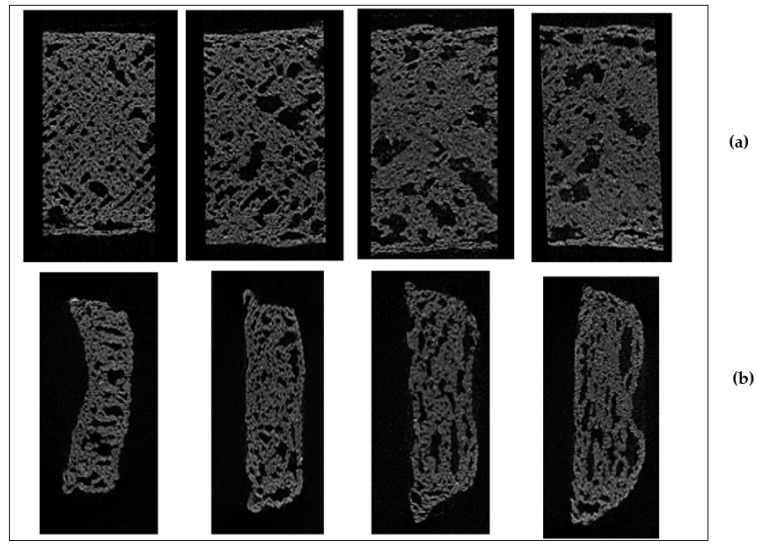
Representative images of (a) top view of reconstructed cross-sectional Micro CT images of baked cookies with 50%, 70%, 90%, and 100% fill density. (b) Cross-sectional view of reconstructed cross-sectional Micro CT images of baked cookies with 50%, 70%, 90%, and 100% fill density.
